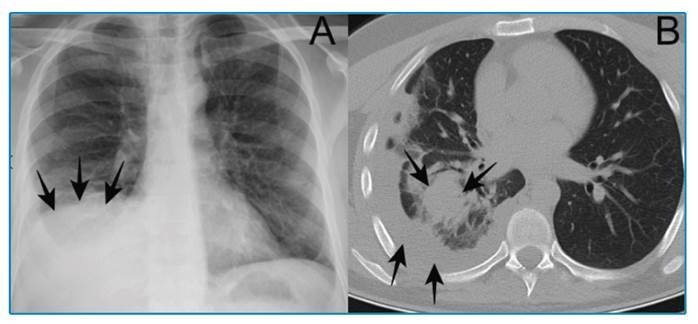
Consolidation and pleural effusion in the right inferior segment of the lung as seen on posteroanterior radiograph examination (A) and thorax CT (B).
